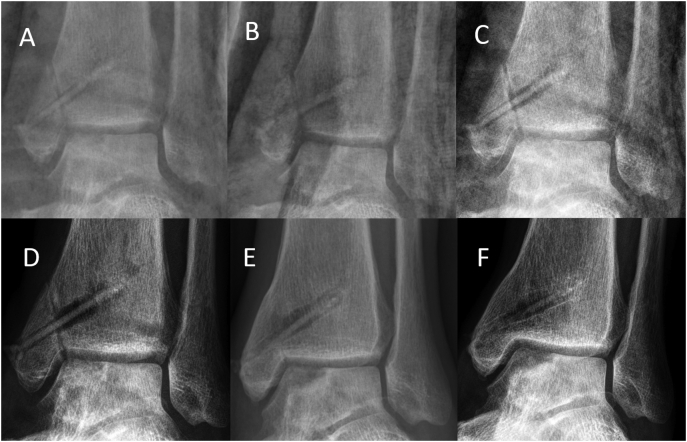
Anteroposterior (AP) radiograph of the ankle. (A) 2 weeks timepoint radiograph, anatomic reduction and stabilization of the medial malleolar fracture with two Magnesium screws; restored joint line of the talus after defect filling with autologous spongiosa. (B) 4 weeks timepoint radiograph (C) 8 weeks timepoint radiograph; visible fracture line at the medial malleolus, small signs of radiolucent zones within the bone surrounding the screws (D) 12 weeks timepoint radiograph, increasing radiolucent area around the screw (E) 24 weeks timepoint radiograph with complete fracture consolidation, decrease of radiolucency within the bone surrounding the screws (F) 52 weeks timepoint radiograph, new bone formation at the radiolucency around the screw, significant degradation of the implant.
